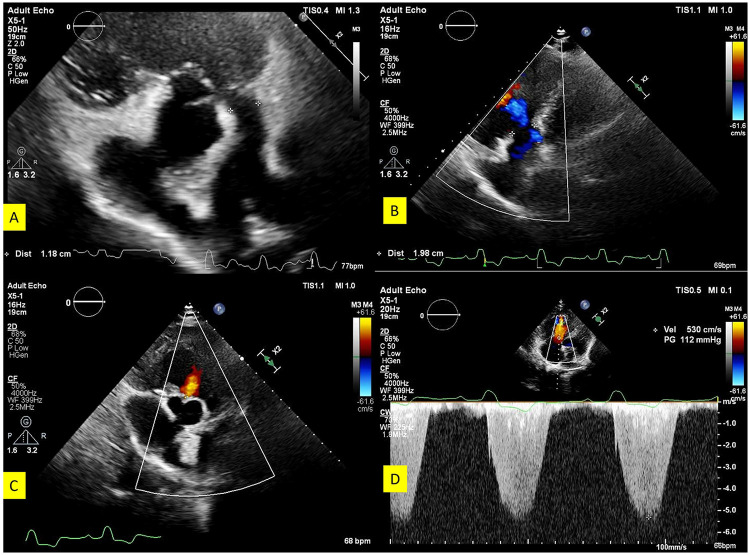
Pre-operative echocardiography diagnosing TOF, (A) main pulmonary artery hypoplasia (1.18 cm), (B) a 2 cm VSD, (C) pulmonic stenosis, (D) tricuspid regurgitation.
